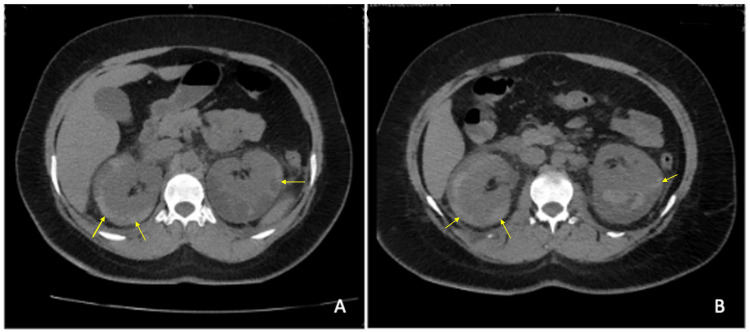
Simple abdominal computed tomography. Liver of 20.5 cm homogeneous density without lesions; right kidney 12.7 cm heterogeneous density, with inflammation of Gerota's fascia; left kidney 11.2 cm heterogeneous density with evidence of inflammation of Gerota's fascia; inflammation of the bilateral perinephric space; thickening of the transverse and descending colon, probable image of diverticula; (A) axial section, upper third of the kidney; (B) axial section of the lower third of the kidney. Yellow arrows indicate inflammation of Gerota's fascia.
